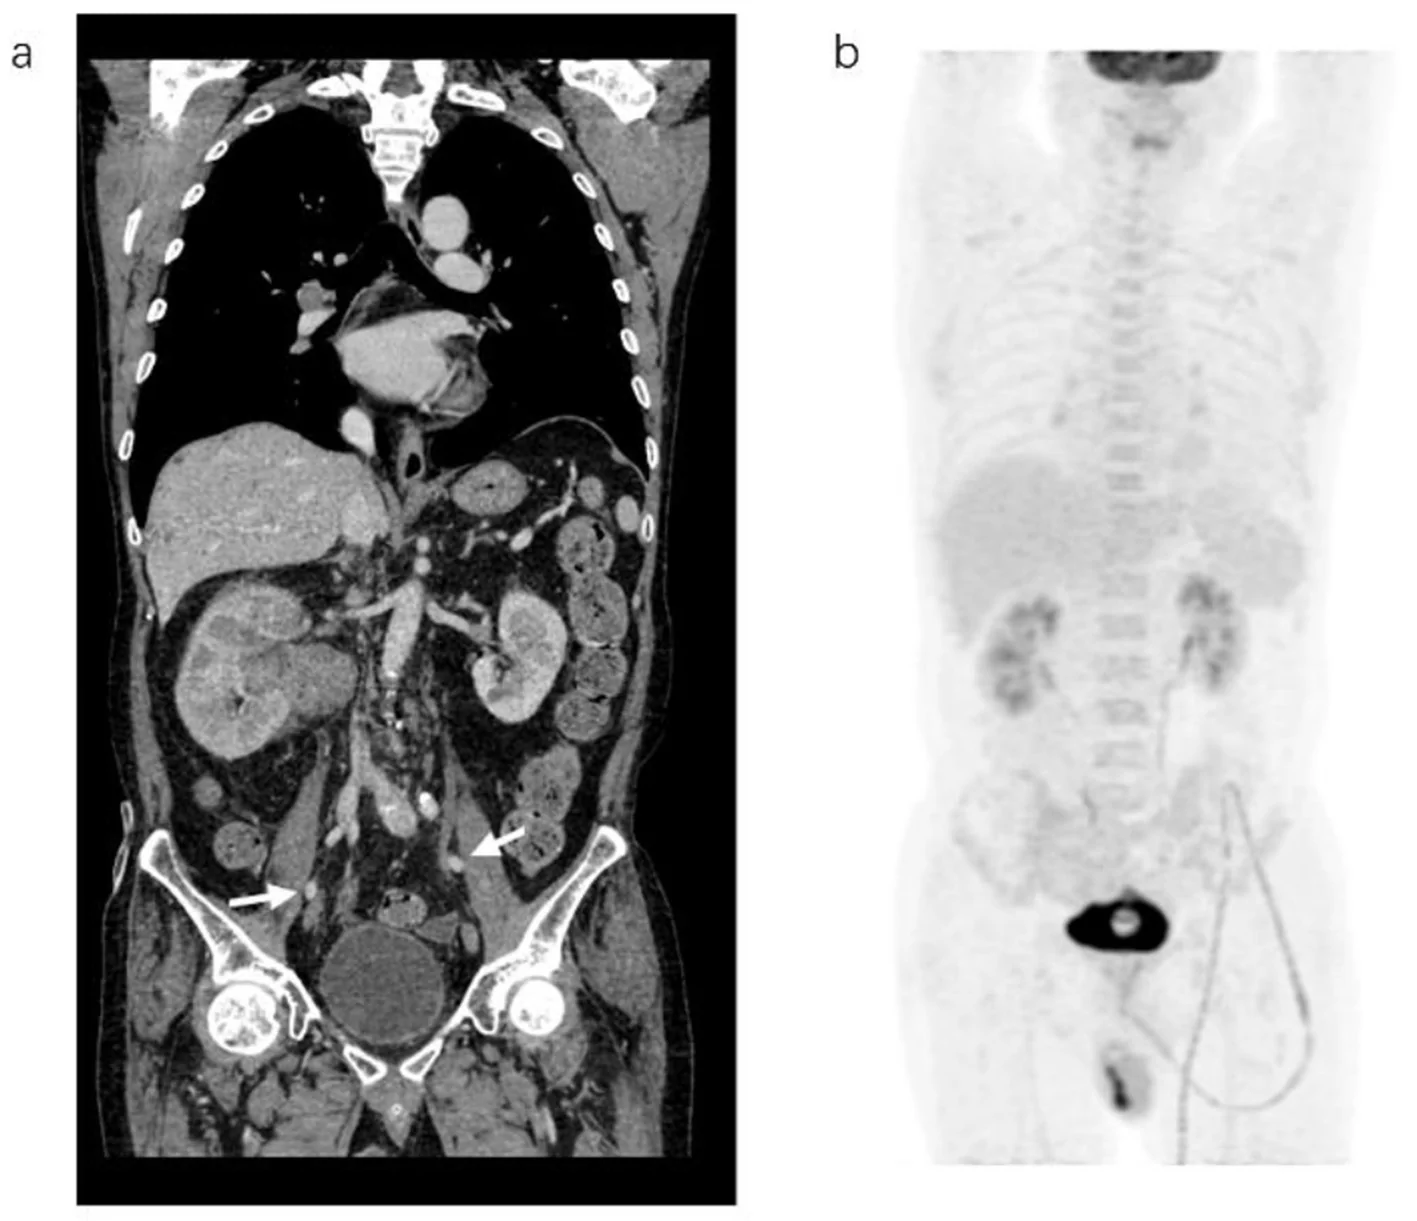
(a) An abdominal CT image of the patient, demonstrating multiple enlarged lymph nodes in the retroperitoneal and pelvic regions. (b) A PET image of the patient, showing no evidence of hypermetabolic neoplastic lesions.
